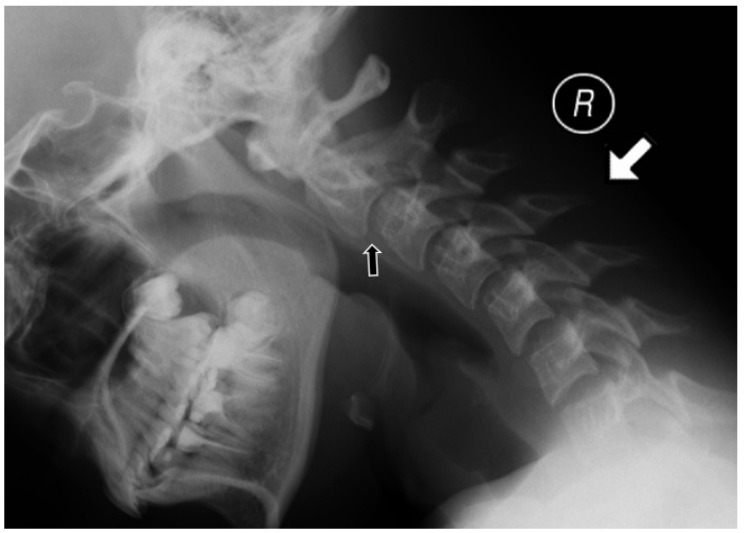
Lateral radiograph in flexion (indicated by white arrow) in a 13-year-old girl showing subaxial subluxation at C2/C3 level (black arrow).
